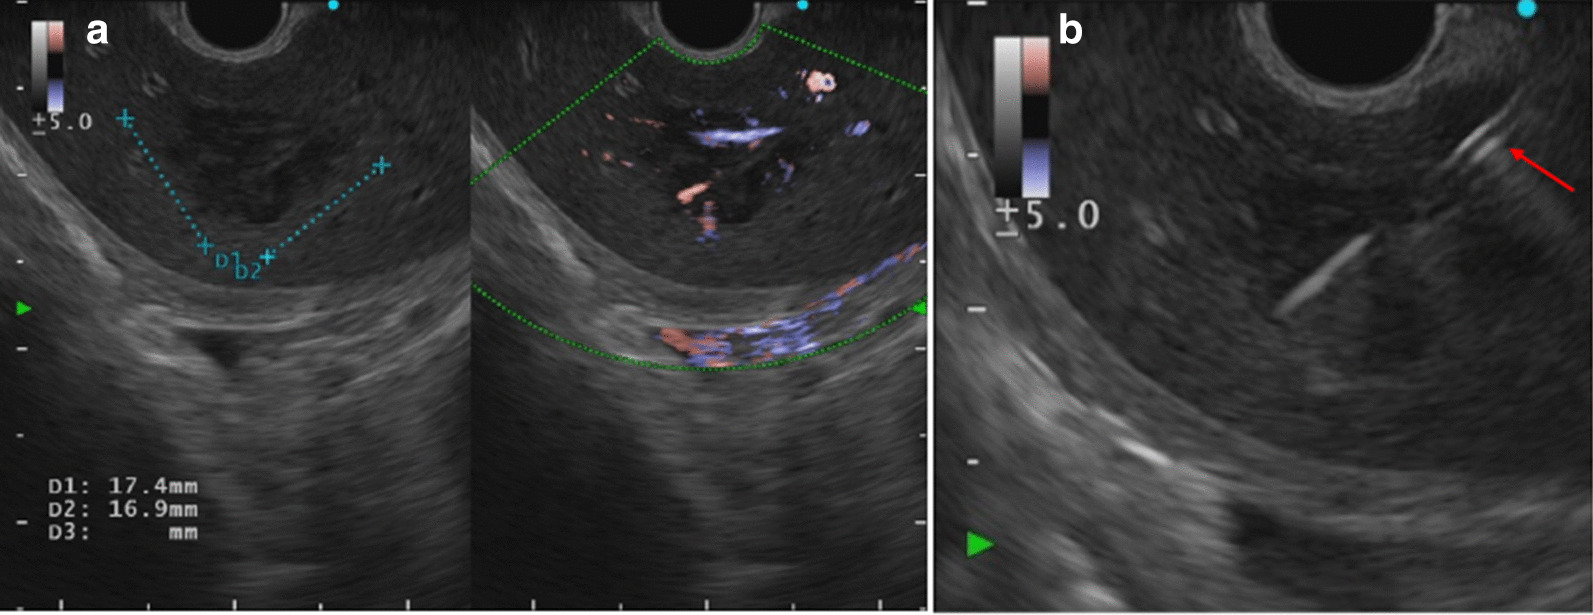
Endoscopic ultrasound findings. a Hypoechoic nodule of the left hepatic lobe (17.4 × 16.9 mm), b endoscopic ultrasound-guided liver biopsy was performed using a 19-gauge needle (red arrow)
